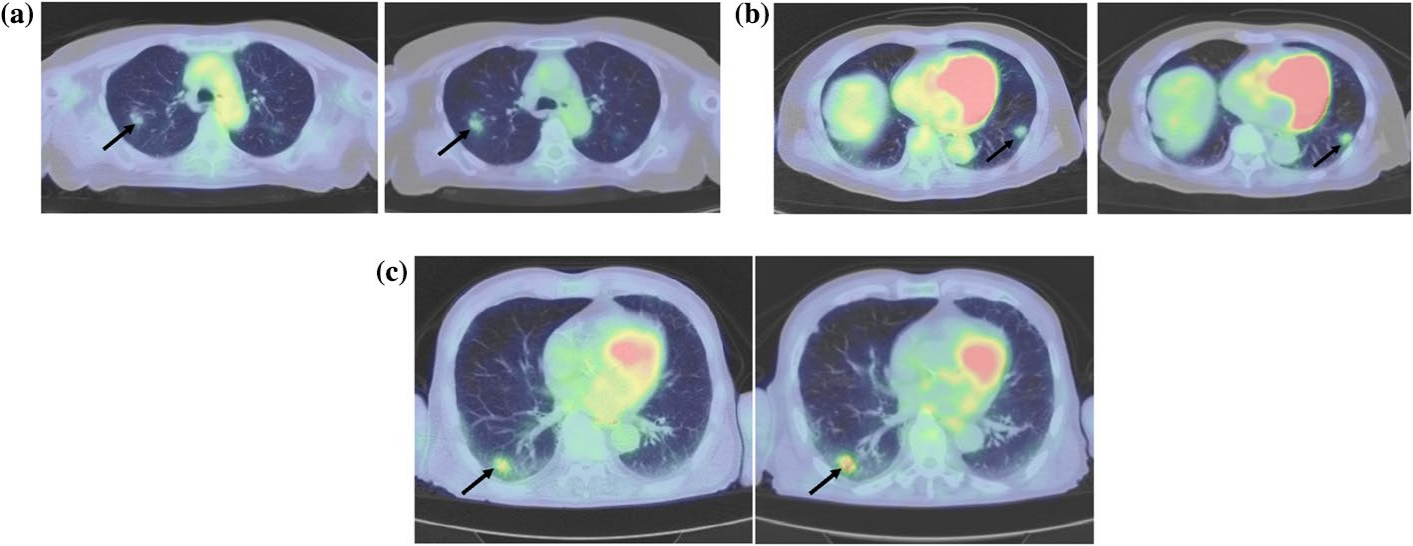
A Adenocarcinoma with no lymph node metastasis. The RI of the primary tumor (arrow) was low at 0.15 (left: SUVmax_e_ 1.3, right: SUVmax_d_ 1.5). B Adenocarcinoma with lymph node metastasis. The primary tumor (arrow) exhibited a high RI of 0.53 (left: SUVmax_e_ 1.7, right: SUVmax_d_ 2.6). C Squamous cell carcinoma with no lymph node metastasis. The primary tumor (arrow) showed a moderate RI of 0.39 (left: SUVmax_e_ 2.3, right: SUVmax_d_ 3.2)
